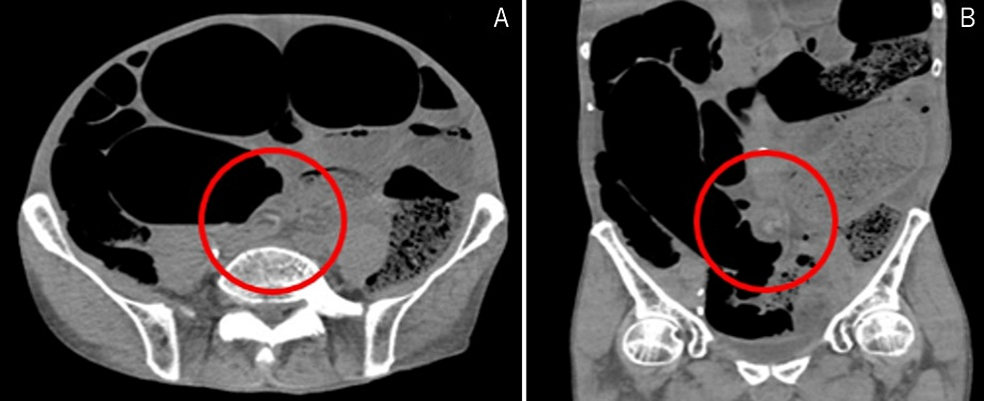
CT findings A and B showed a caliber change of the sigmoid colon, suggesting a sigmoid volvulus (red circle). CT: computed tomography
